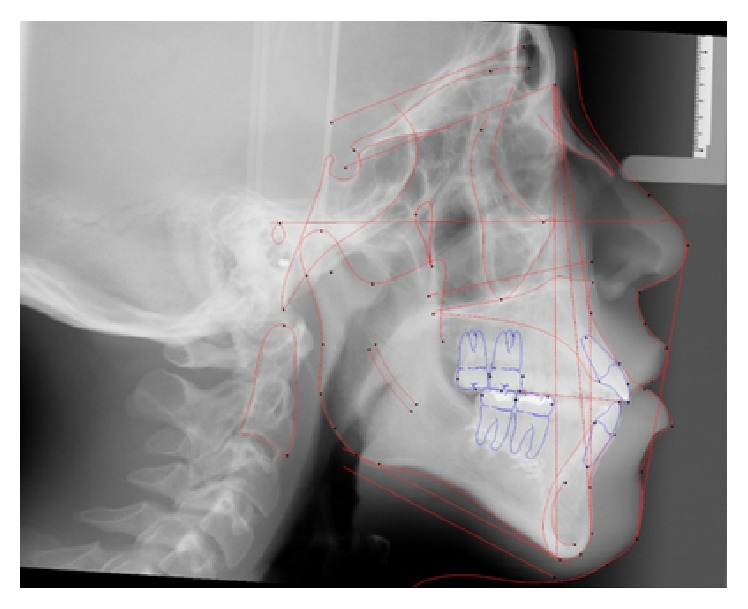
Stage 2: Orthodontic Treatment Final Cephalometric Radiograph.
